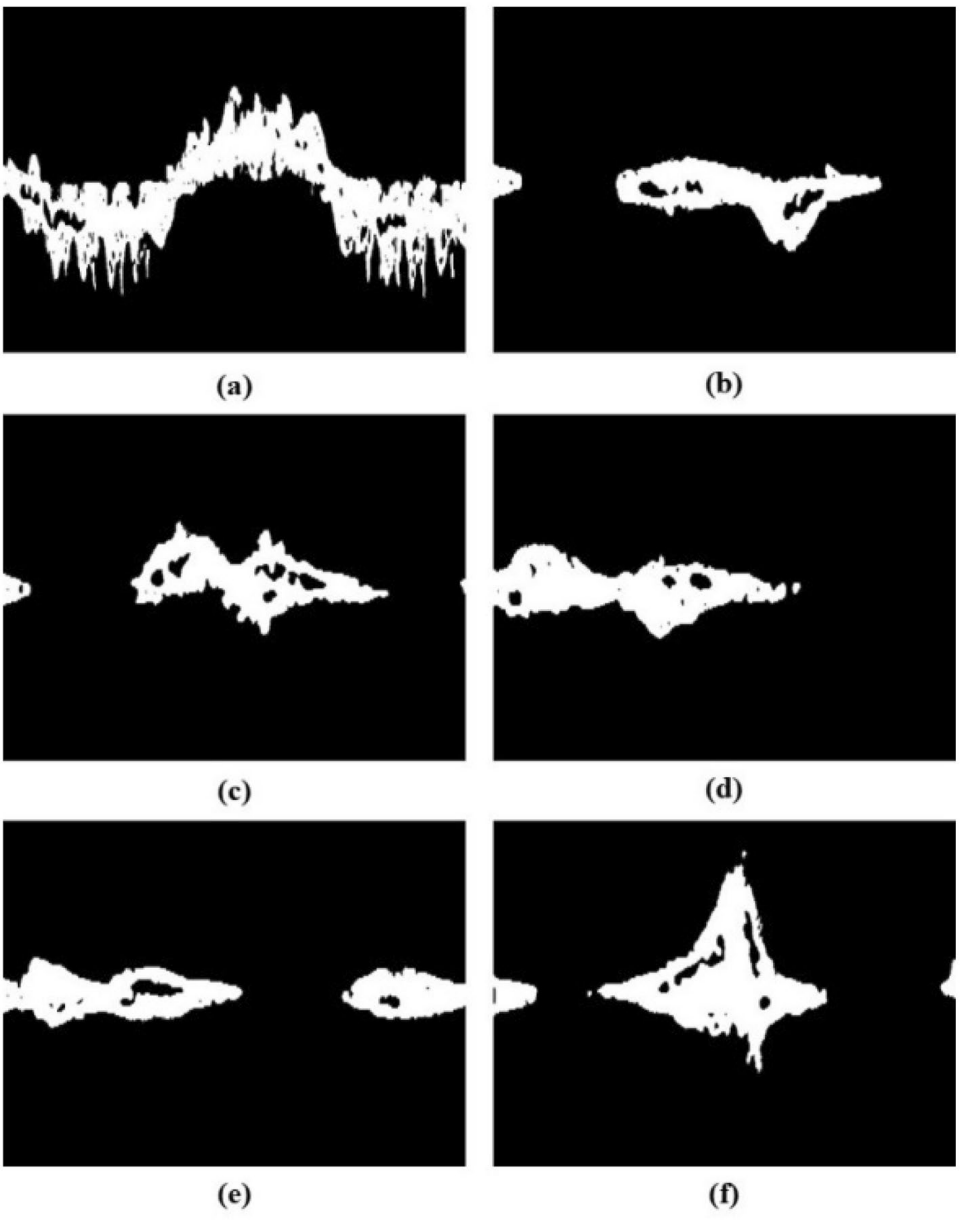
The binary mask of typical samples of the dataset. (a–f) represent activities A1 ~ A6 micro-Doppler spectrograms.
